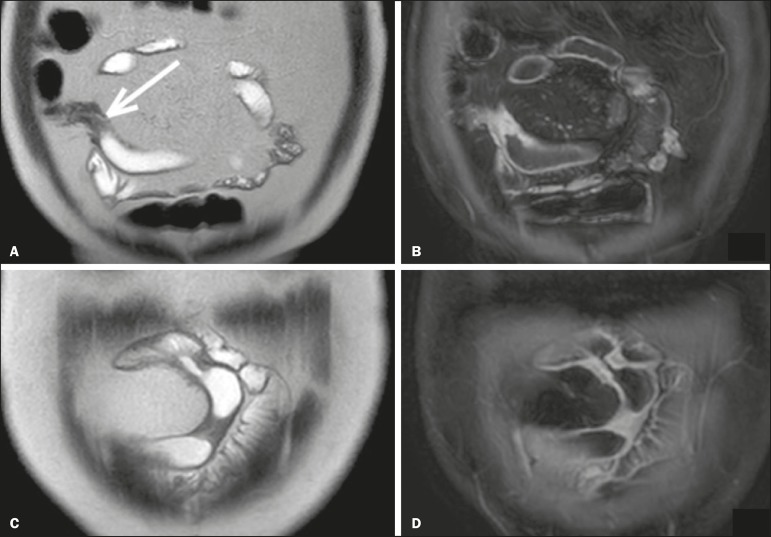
Coronal T2-weighted MRE sequence (A,B) and coronal, paramagnetic contrast-enhanced, fat-saturated T1-weighted MRE sequence (C,D) showing signs of fibrosis and mild stenosis (arrow) in the distal ileum (A,C) and inflammatory activity in the medium ileum (B,D). Also note the use of the biphasic oral contrast (polyethylene glycol) distending the intestinal lumen, with high signal intensity in the T2-weighted sequence and low signal intensity in the T1-weighted sequence.
